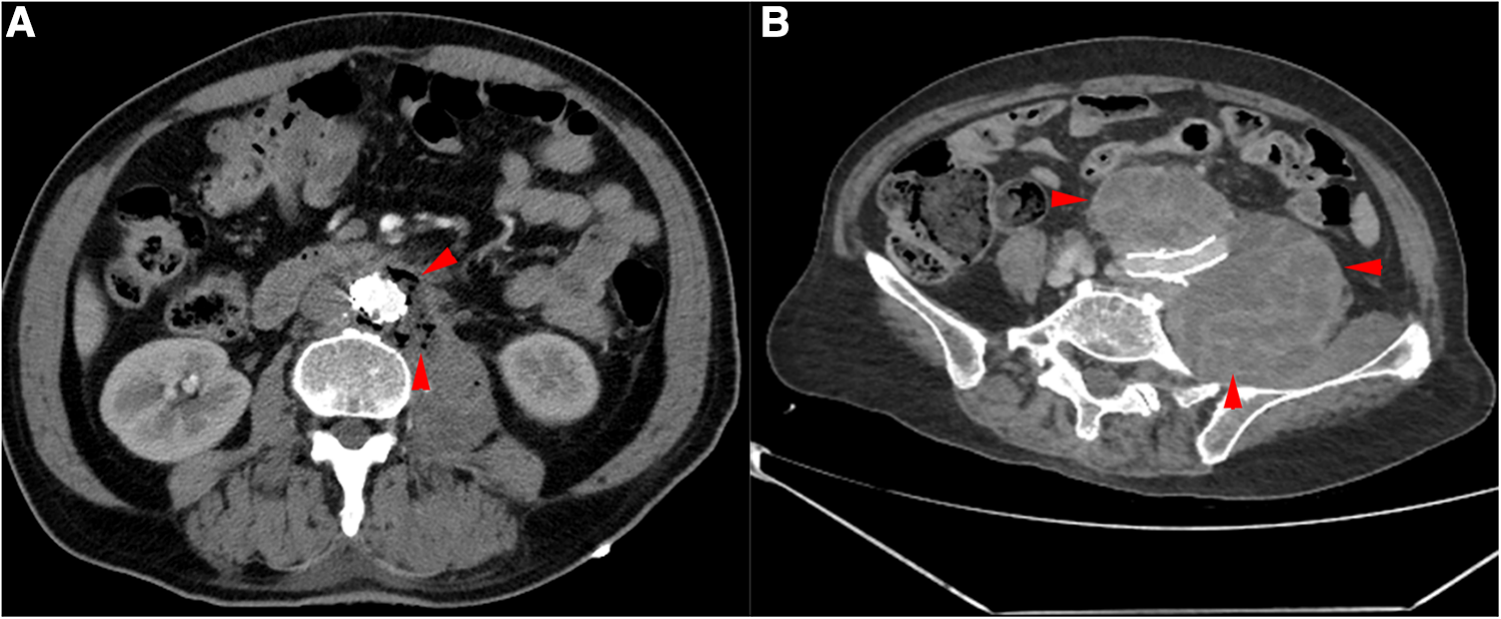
CTA of the patient's aorta. (A) Red arrows indicate the presence of gas around the aorta. (B) Red arrows indicate haematoma around the common iliac artery.
